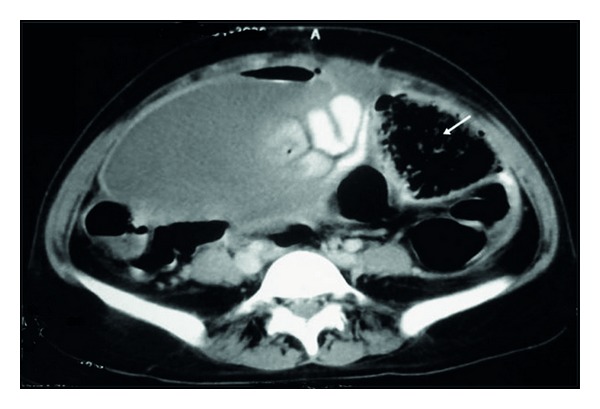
CT of abdomen showing a large fluid collection and another heterogeneous collection with air trapped within, on the left side [gossypiboma].
